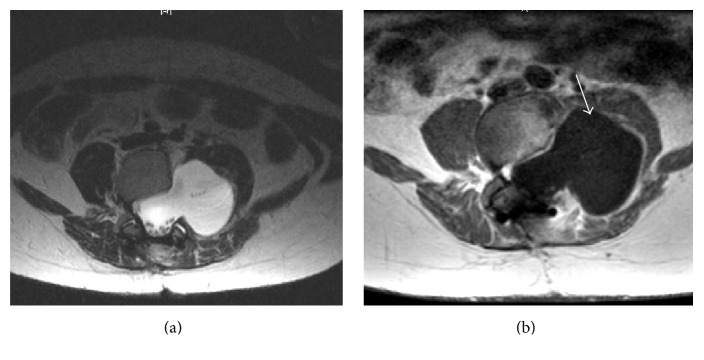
One year after the second operation an axial T2 and T1-weighted postgadolinium MRI images disclosed a pseudomeningocele with roots compression and displacement of the psoas muscle (white arrow).
